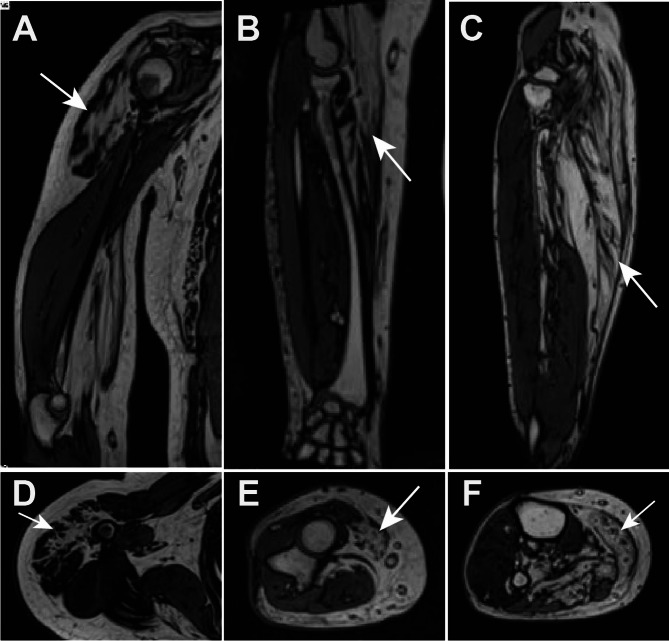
MRI of the right proximal and distal arm in patient No.5 revealed increased signal intensity and fatty infiltration (A and B) and MRI of the lower leg in patient No.5 revealed significant fatty replacement along with muscle atrophy in the posterior compartments of lower leg muscles. (C), coronal axial MRI image of the right proximal and distal arm and lower leg muscles in patient No.5. (D. E and F)
